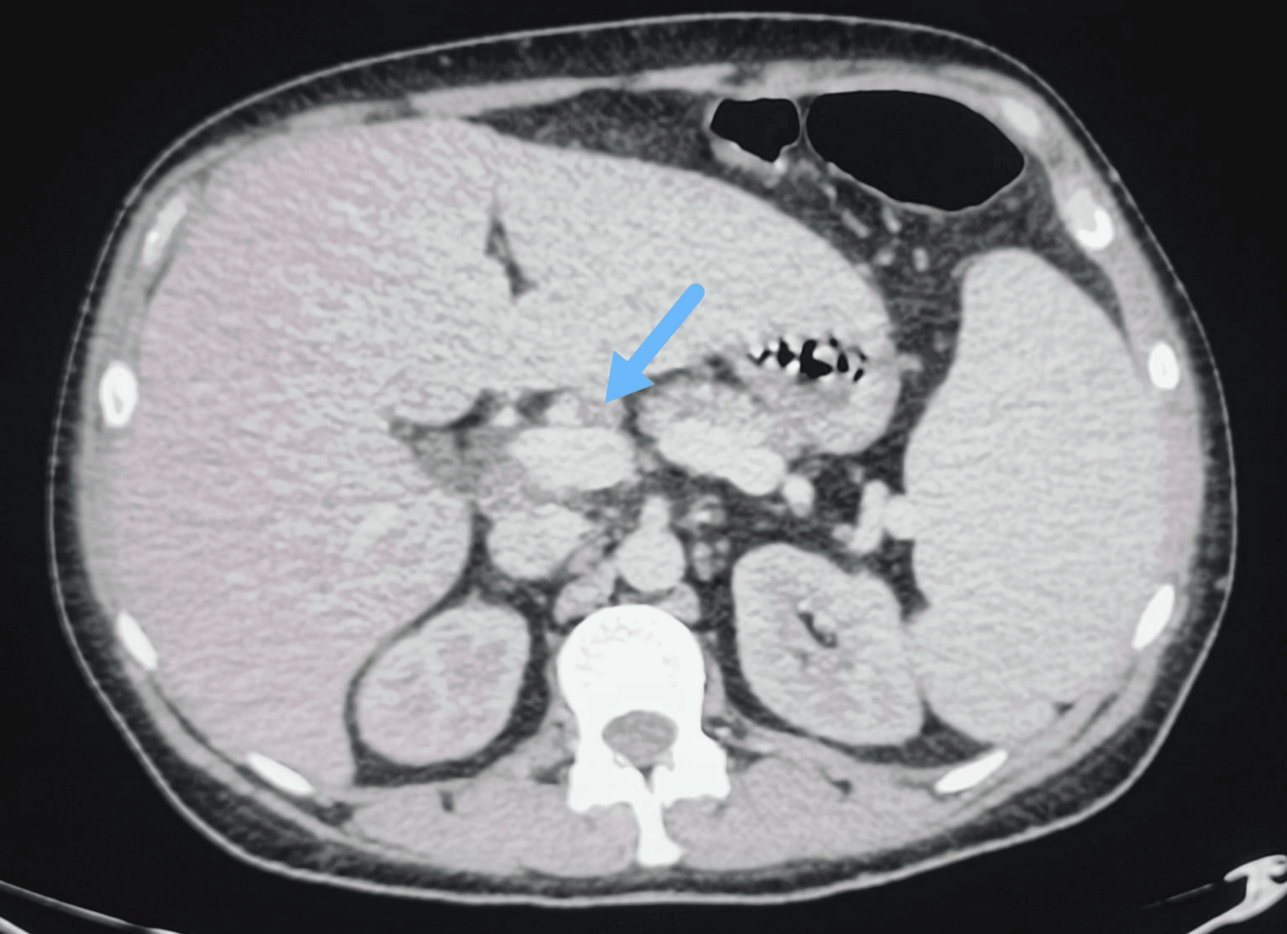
Abdominal CT scan demonstrating perihepatic lymph nodes The blue arrow indicates a perihepatic lymph node.
